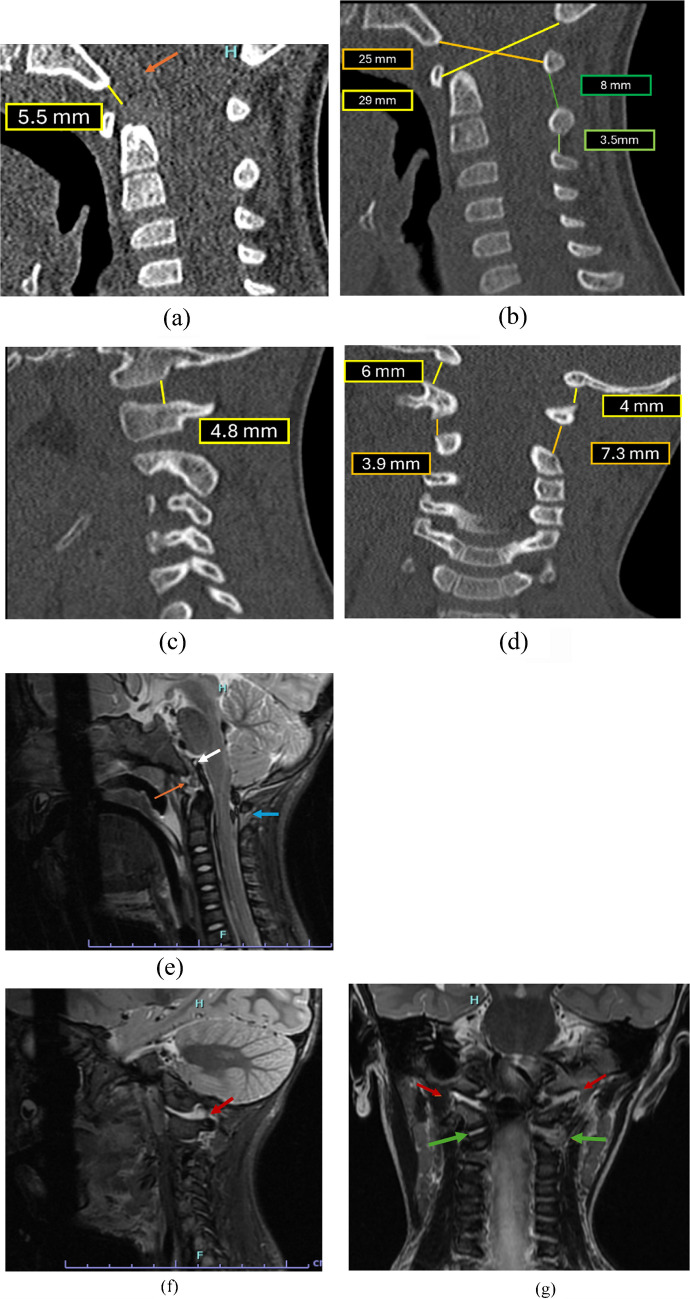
(a, b) Sagittal CT images demonstrate normal BBasion Cartilaginous Dens Interval  (BCDI) measuring 5.5 mm [N<5.6 mm] and Powers ratio which measured 0.8 [N<0.9]. A subtle retroclival hyperdensity is noted (*orange arrows*), though characterization is limited on CT. The interspinous distance ratio measures 2.3 which is also within normal limits [N<2.5]  (c, d) Sagittal and coronal CT images reveal asymmetry of the occipital condyle–C1 interval as shown in the right parasagittal region measuring 4.8 mm  and on the coronal images measuring 6 mm on the right and 4 mm on the left (*N*<2.5 mm on sagittal and <3.5 mm on coronal). The atlantoaxial interval is also increased on both sides measuring 3.9 mm on the right and 7.3 mm on the left (N<3.9 mm). Subsequent MRI findings: (e) sagittal T2 fat saturated images of the cervical spine  demonstrates elevation of the tectorial membrane (*white arrow*) with thin interposed fluid layer between the membrane and clivus, without complete detachment. There is edema in the basion-dens interval (*orange arrow*) and in the interspinous region between C1 and C2 (*blue arrow*) without significant widening indicative of soft tissue edema. (f) Parasagittal T2 fat saturated images revealed T2 hyperintensity within the occipital condyle–C1 (*red arrows*) with V shaped widening. (g) Coronal T2 fat saturated images confirm widening of the occipital condyle–C1 interval on both sides with fluid in these regions (*red arrows*). There is widening with fluid also seen in bilateral atlantoaxial intervals (*green arrows*), consistent with craniocervical distraction injury
